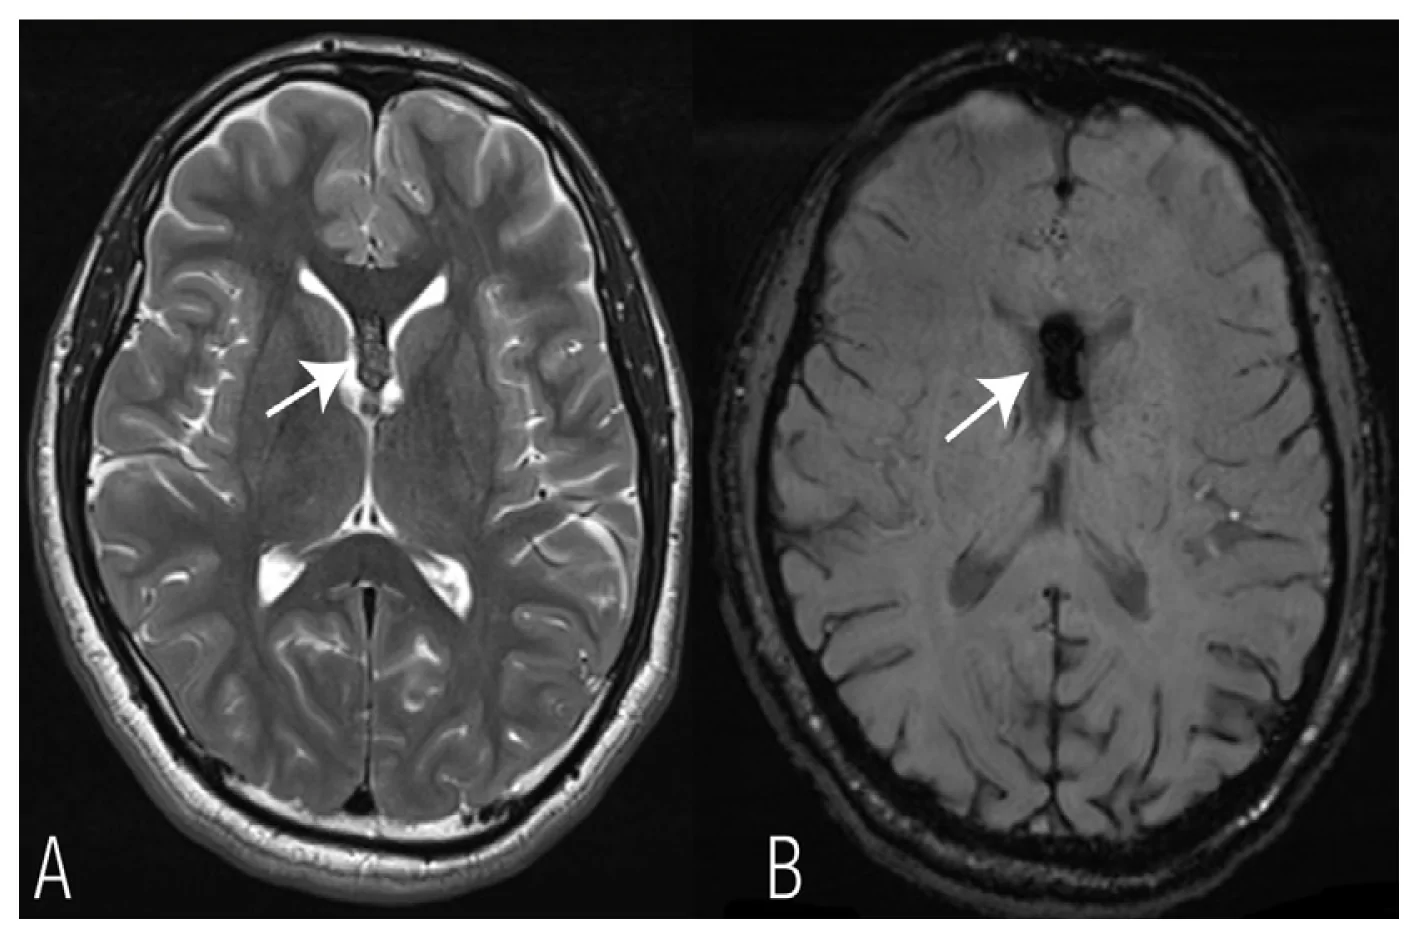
Magnetic resonance imaging at follow-up after 18 months showing (A) an interval reduction in the size of the lesion with a siderotic rim on an axial T2-weighted image (arrow) and (B) susceptibility effects due to old blood products in susceptibility-weighted imaging (arrow).
